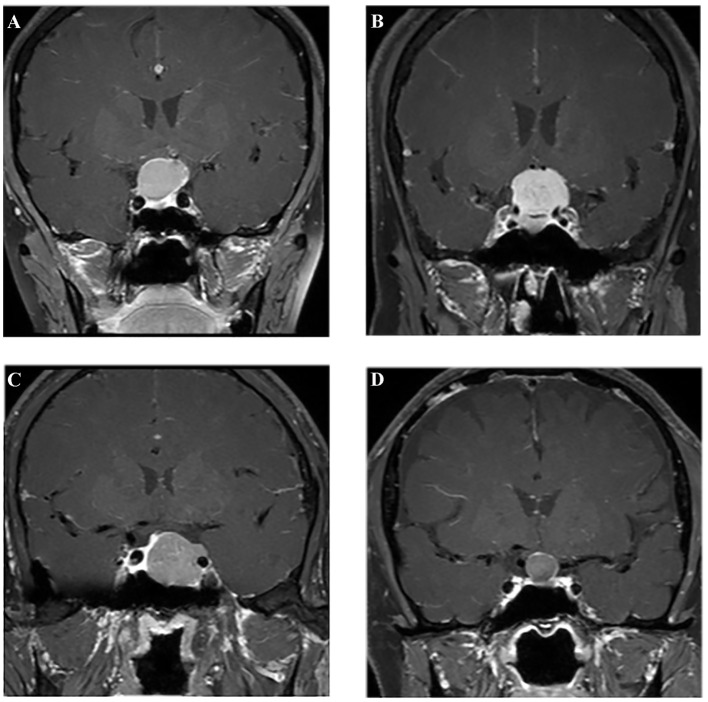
Examples of different lesions on contrast-enhanced T1-weighted image. (A) Craniopharyngioma; (B) meningioma; (C) pituitary adenoma; (D) Rathke cleft cyst.
